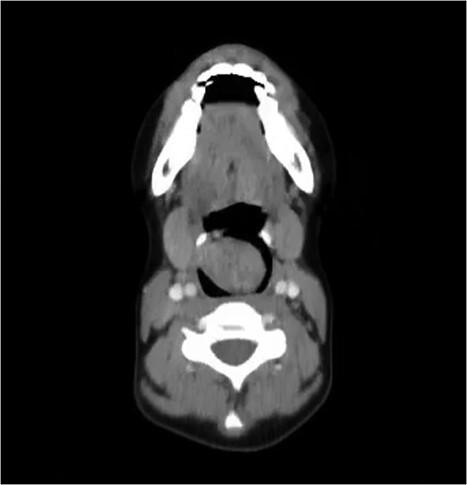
CT of the neck with axial cut showing the schwannoma occupying the larynx.
